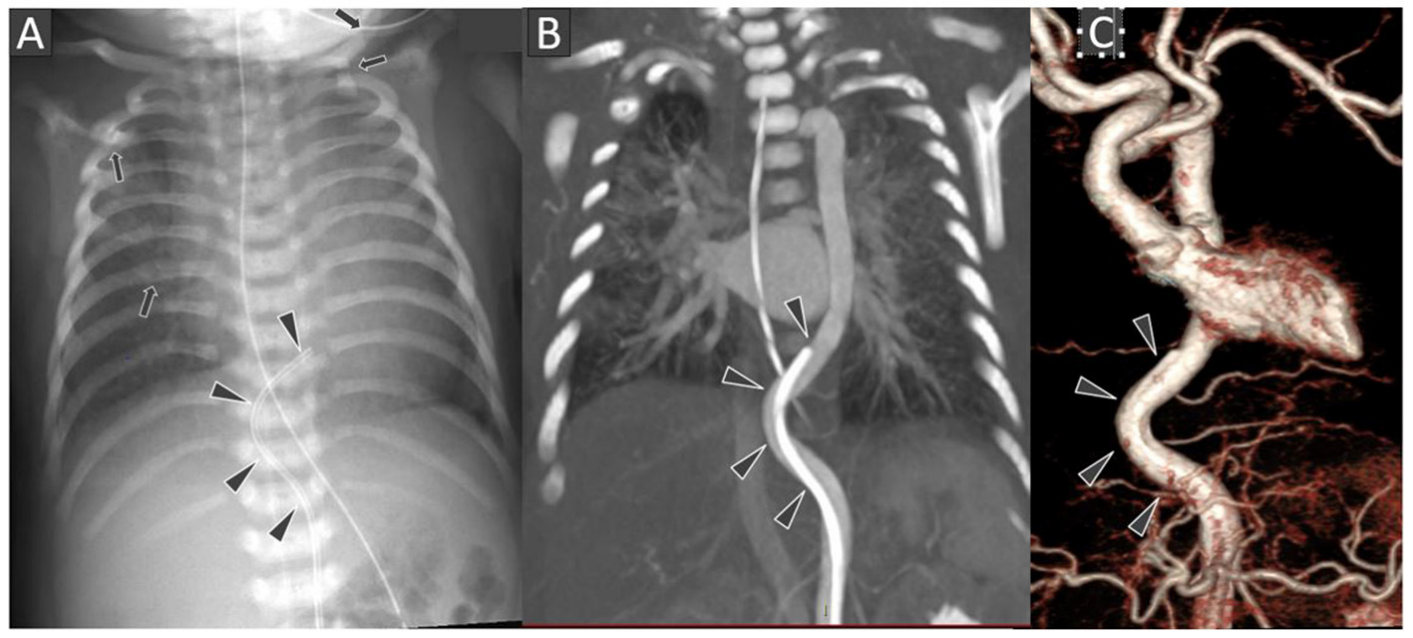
Frontal chest radiograph (A) shows the tip of the umbilical arterial catheter (UAC) at the level of T8 vertebral body with curved configuration of its terminal portion (black arrowheads). Several rib and left clavicular fractures (black arrows) are also noted in the bony thoracic cage. Chest CT Angiography coronal multi-planar reconstruction image at the level of thoraco-abdominal aorta (B) and 3D surface shaded display image of the thoraco-abdominal aorta (C) showing the arterial tortuosity with distal end of the UAC location in descending thoracic aorta.
